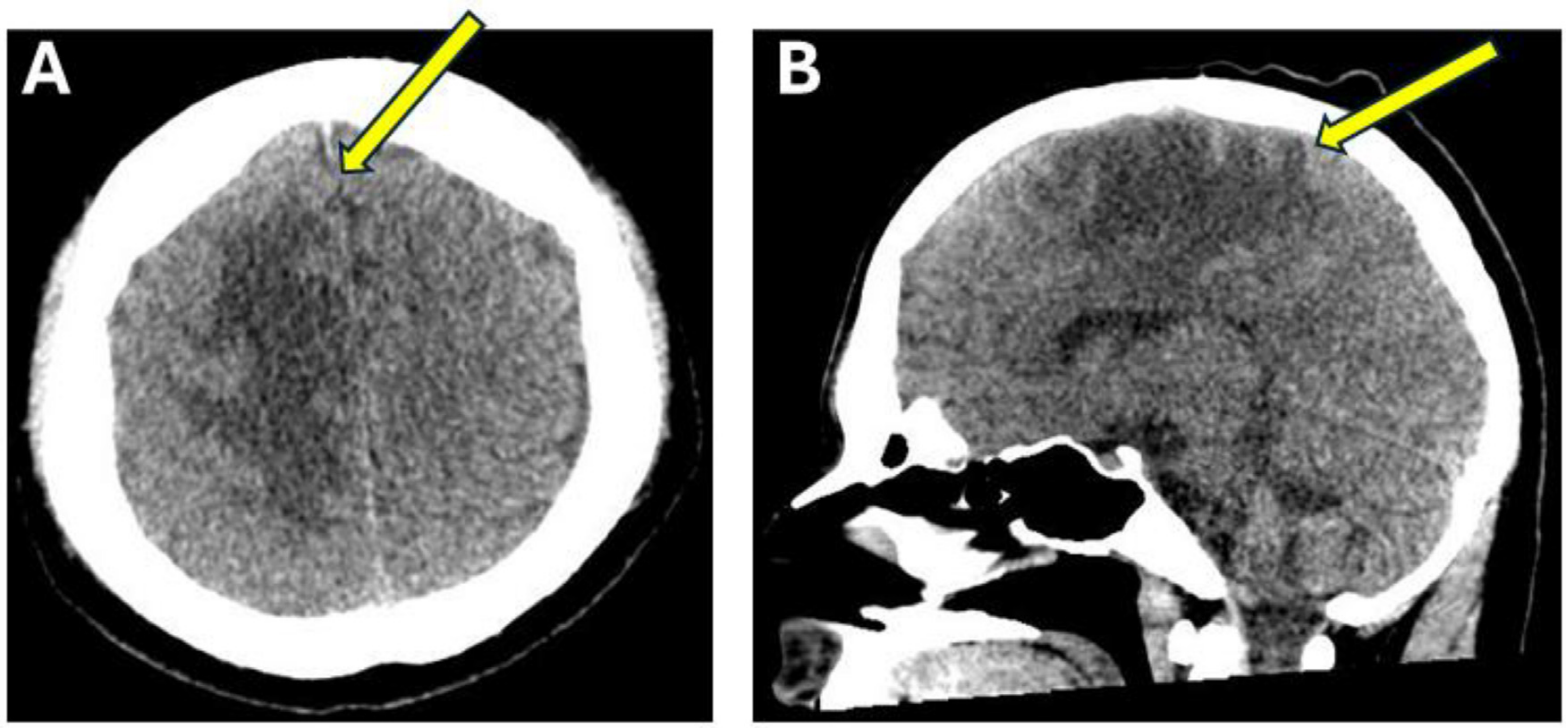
Head CT preceding the MRI obtained after the patient was transferred to the large academic center. Axial (A) and sagittal (B) head CT images demonstrate a large area of vasogenic edema (arrows) throughout the paramedian right frontal and parietal lobe.
